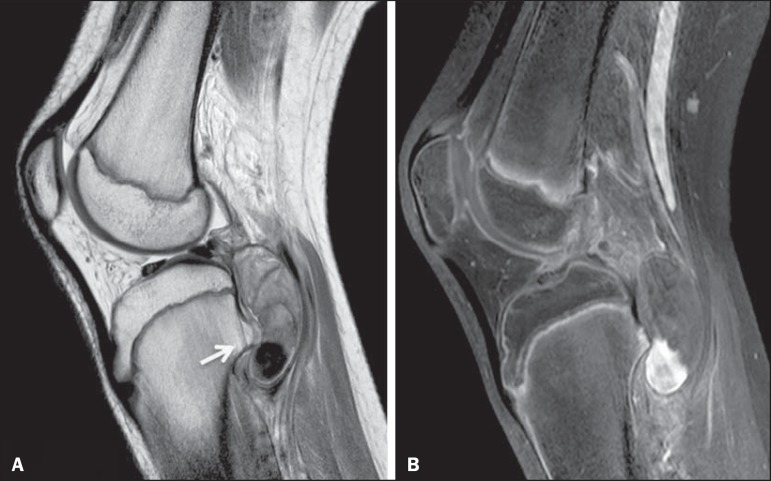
Popliteal artery pseudoaneurysm. Sagittal PD-weighted MRI sequence
